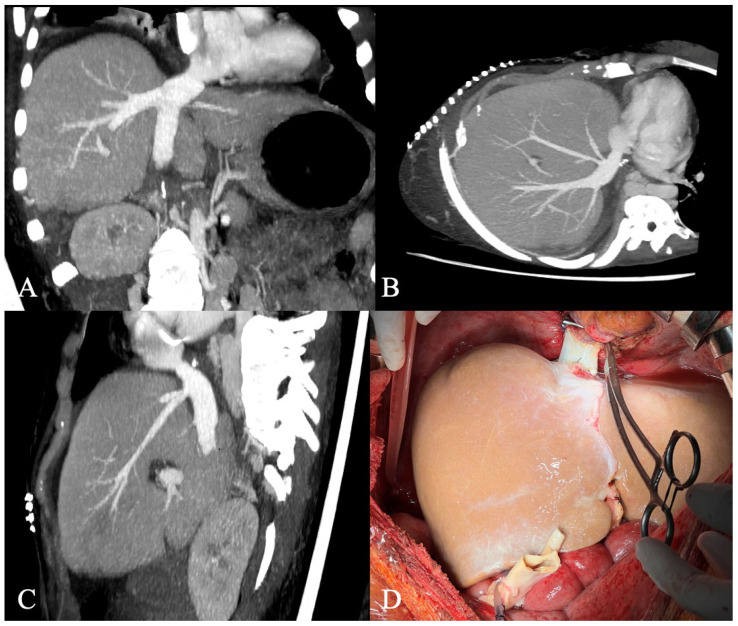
Posttransplant CT scans (A–C) and intraoperative picture of the IVC-atrial anastomosis (D). The pretransplant recipient CT scans show anastomosis of the donor IVC to the recipient’s right atrium (coronal plane, (A); axial plane (B), sagittal plane (C)). CT, computed tomography; IVC, inferior vena cava.
